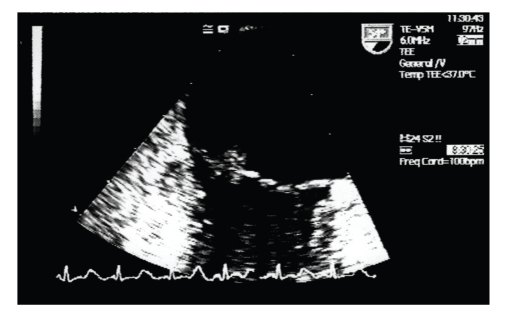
Transesophageal echocardiography shows an echodense structure (0.7 cm by 1 cm) on the atrial aspect of the anterior mitral leaflet.
